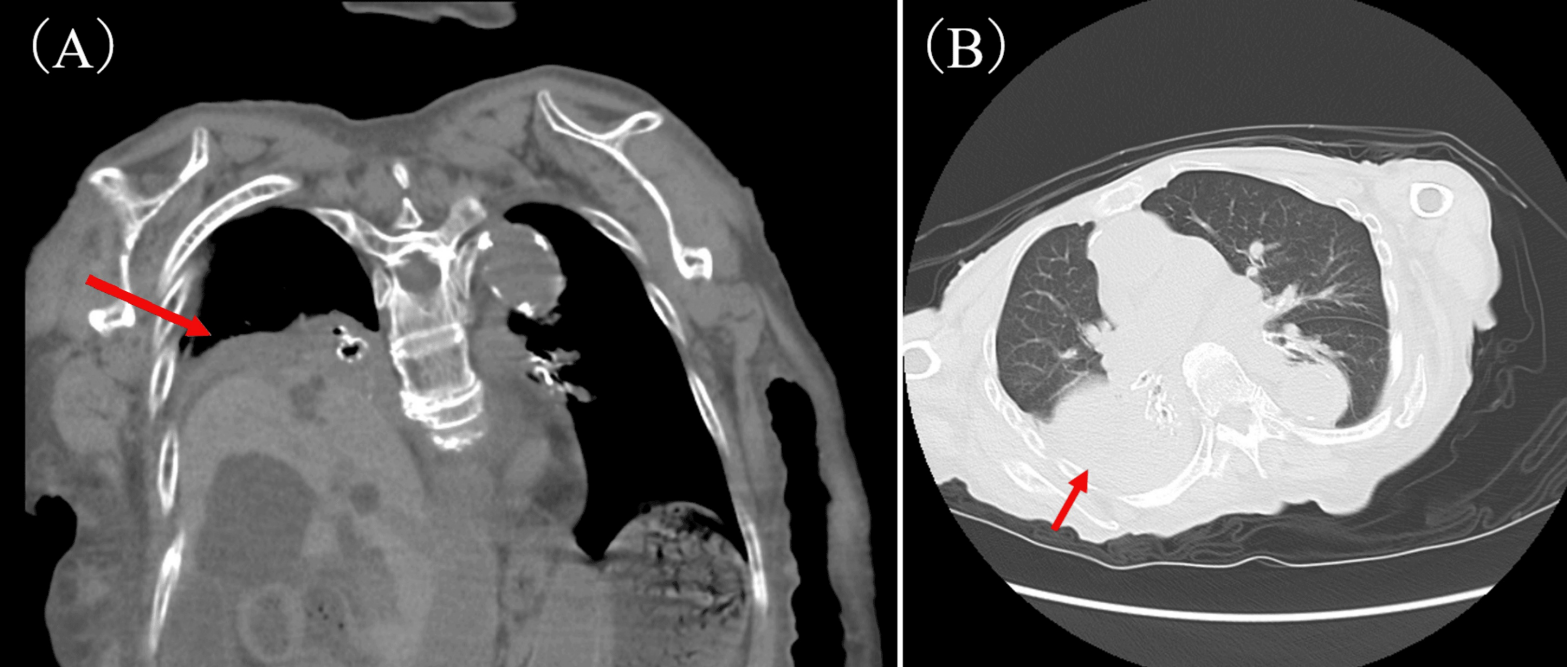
Thoracic CT scan Chest CT scan (A: coronal section; B: axial section) showing right diaphragmatic elevation, atelectasis, and a small pleural effusion (arrows).
